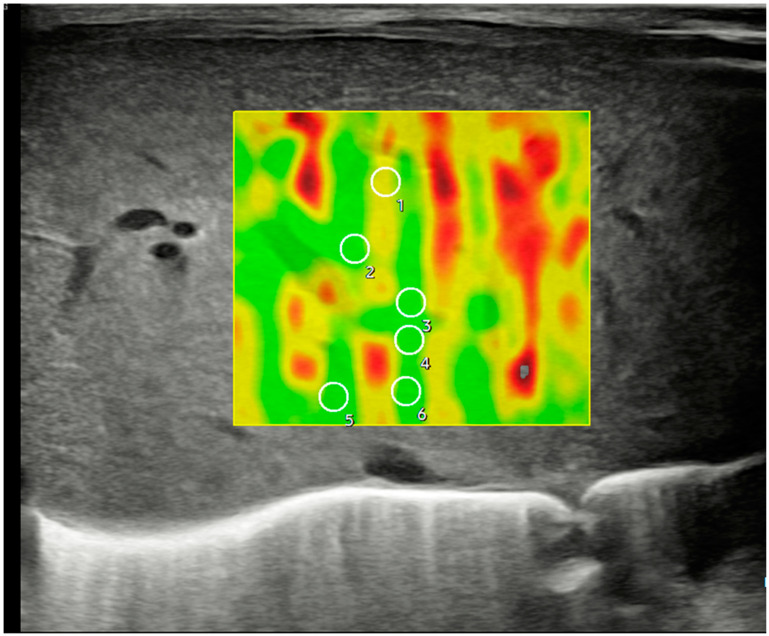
Ultrasonographic example of a hepatic axial section in segment IV with sample acquisition using 2D-SWE and a linear probe, following the same methodology described in Figure 2.
